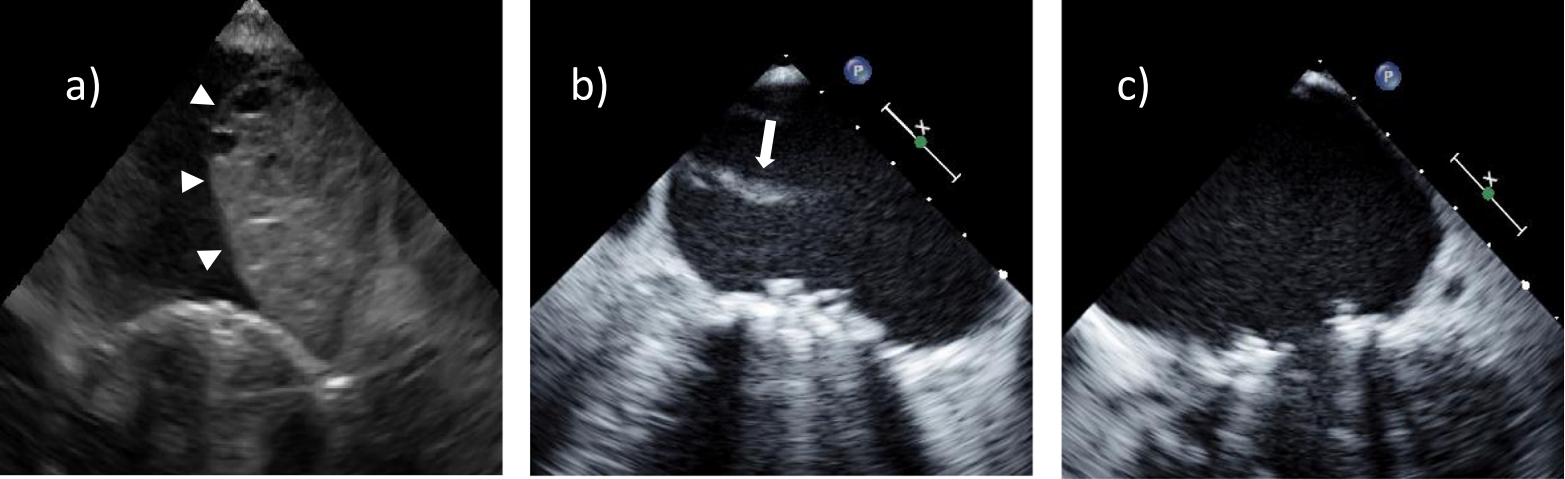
Serial transesophageal echocardiography. a Intraoperative transesophageal echocardiography revealing an oval hypoechoic appearance (white arrowheads) extending from the posterior annulus of the mitral valve to the posterior wall of the left atrium. b Transesophageal echocardiography on postoperative day 2 revealing the vanishing atrial mass and the remaining broken piece of the endocardium fluttering in the atrium (white arrow). c Transesophageal echocardiography on postoperative day 6 revealing the complete disappearance of the left atrial dissection
